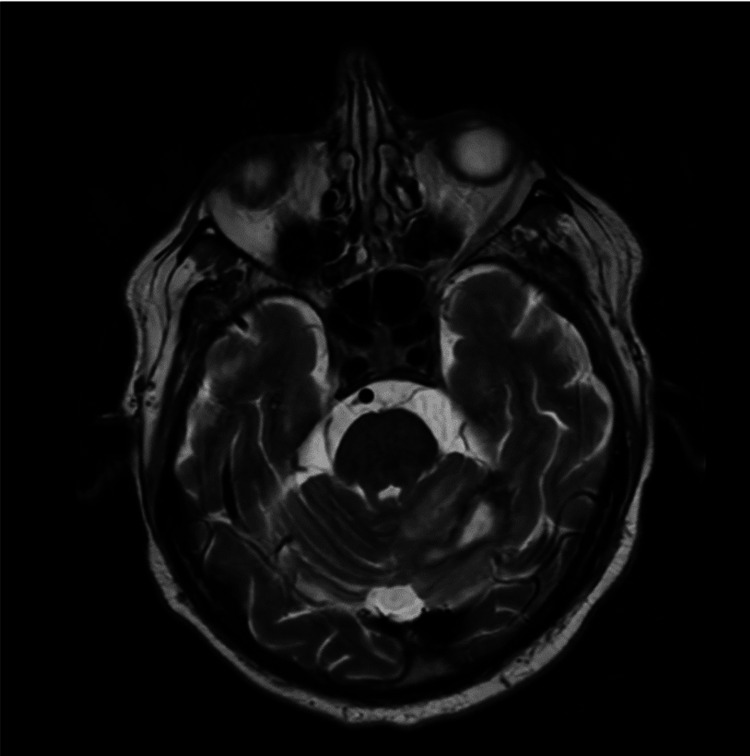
Outcomes of the MRI of the brain in October 2023, indicating a notable reduction in the size of the lesion situated in the left cerebellum.
